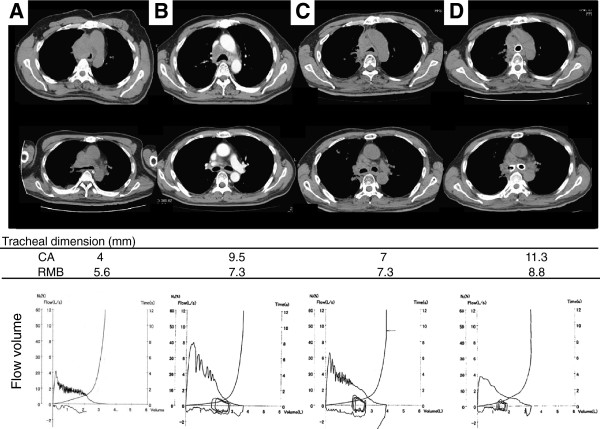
Sequential findings on thoracic CT and flow-volume loops. Sequential findings on thoracic CT and flow-volume loops from the day of admission (day1) to the day224. At day1 (Figure 1-A) showed a severe narrowing of the trachea,to approximately 4mm in diameter with moderate stenosis (5mm) in the right main bronchus, which accompanied by saw-tooth sign with trapezoidal shape on flow-volume loops. At day60 (Figure 1-B) revealed the improvement of the limitation of expiratory flow with the disappearance of the saw-tooth sign or trapezoidal shape as well as the amelioration of the stenosis both in the central airway and right main bronchus. At day207 (Figure 1-C), flow-volume loops showed the reproduction of the saw-tooth sign together with the progression of stenosis in the central airway (7mm), but not in the right bronchus. At day224 (Figure 1-D), after completion of inserting stent, saw-tooth sign completely disappeared.
